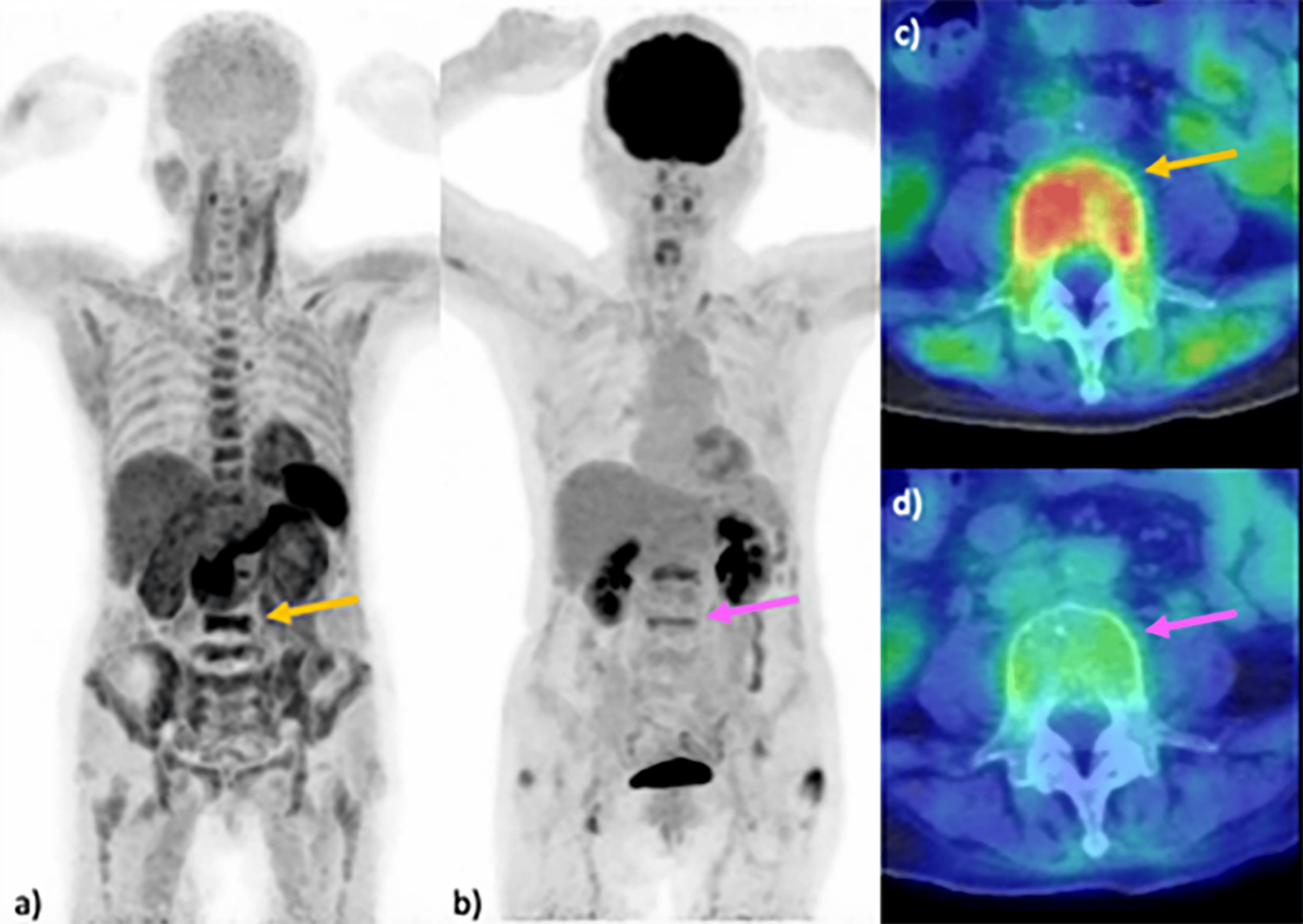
Comparison of PET imaging in MM: 11C-acetate versus 18F-FDG Imaging results from a 68-year-old woman with MM are presented. MIP images reveal multiple lesions in the vertebrae, including the L5 vertebral body (arrow) and pelvic bones, identified using 11C-acetate PET (a). Lesions in the lumbar vertebrae, including the L5 vertebral body (arrow), are detected by 18F-FDG PET (b). An axial fused PET/CT image utilizing 11C-acetate at the L5 vertebra demonstrates increased uptake in the vertebral body (SUVmax 4.0) (arrow) (c), in contrast to the relatively weak uptake observed in the 18F-FDG PET/CT image (SUVmax 2.0) (arrow) (d). FDG, fluorodeoxyglucose; MIP, maximum intensity projection; MM, multiple myeloma; SUV, standardized uptake value
